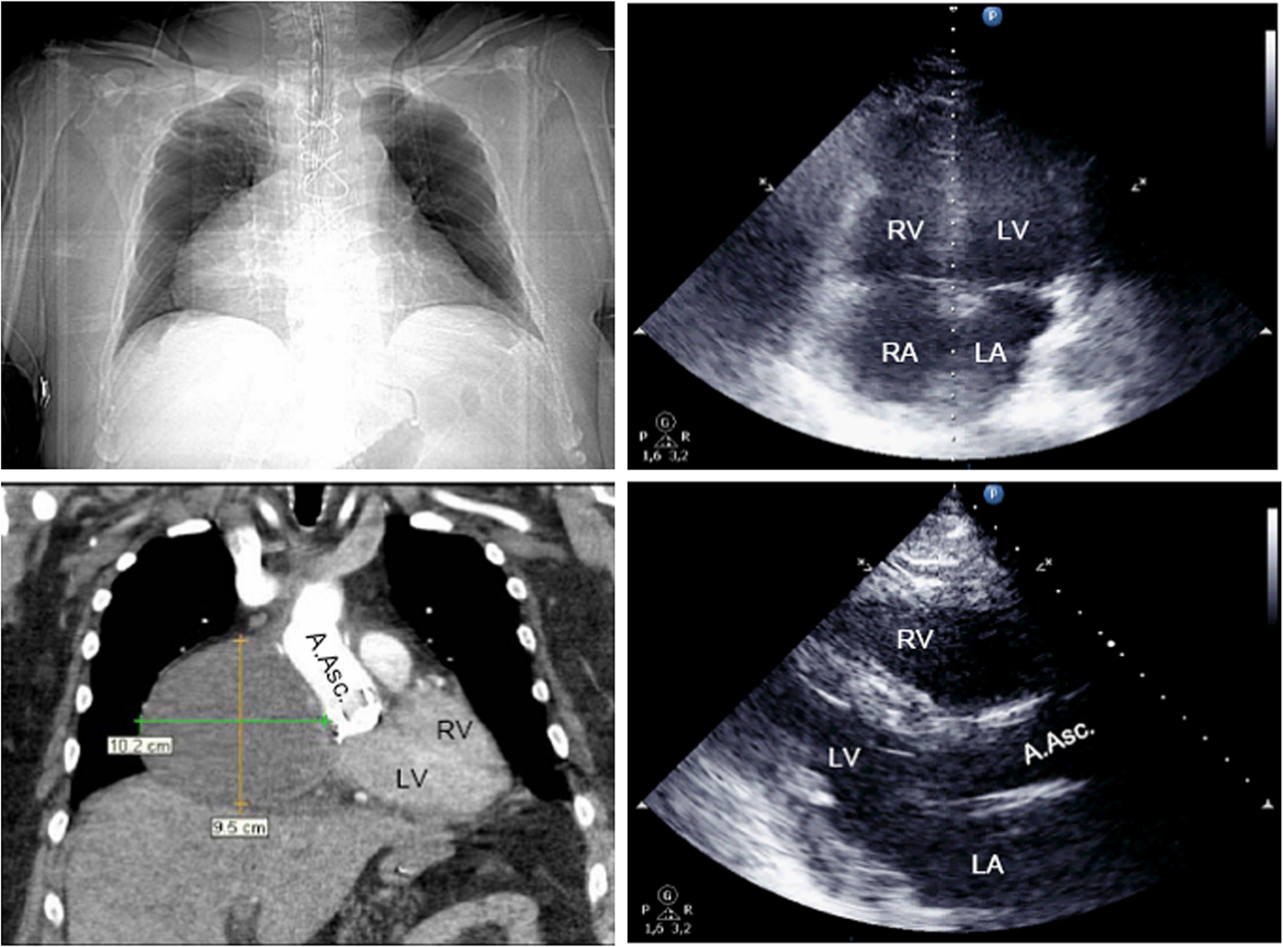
Patient with cardiac tamponade on day 15 after ascending aortic replacement. Right atrial hematoma (102 × 95mm) not detected by transthoracic echocardiography (B-mode: apical 4-chamber-view *right above*, parasternal long axis *right below*). Thoracic CT-imaging with contrast medium, arterial phase (topography scan *left above*, coronary view, soft tissue window *below left*). Diagnostic imaging initiated after collapse necessitating cardiopulmonary resuscitation. LV left ventricle, RV right ventricle, LA left atrium, RA right atrium, A.Asc. ascending aorta.
